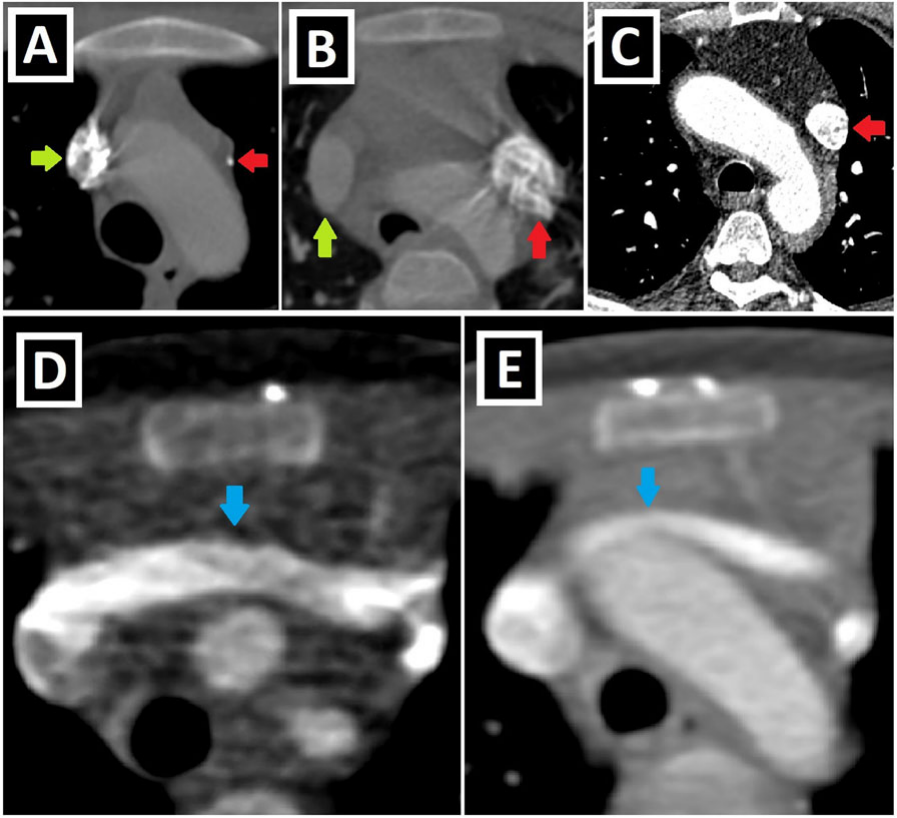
Size of PLSVC, presence of RSVC/bridging vein. Axial CT images of different patients. a PLSVC (red arrow) with a significantly smaller caliber than RSVC (green arrow). b PLSVC (red arrow) having similar sizes to RSVC (green arrow) in a patient with double SVC. c Isolated PLSVC (red arrow) in a patient without any accompanying cardiac anomaly. d, e Prominent (d) and relatively less prominent (e) bridging veins (blue arrows) compared to bilateral SVC diameters
